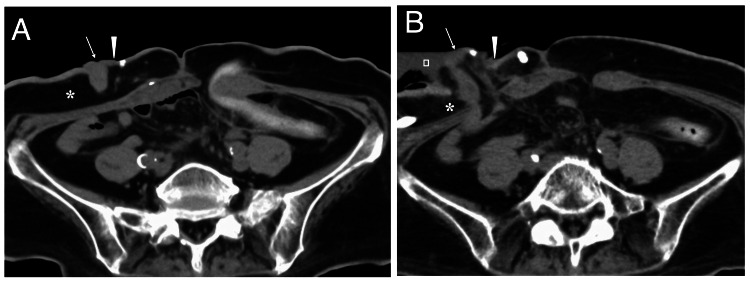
Computed tomography (CT) of and around the ileostoma A: CT showed that subcutaneous fat was thick as 3 cm (asterisk), and the top of the ileostoma (arrow) was located at the same level of the ileostoma base (arrowhead) and the surrounding skin before reoperation; B: although watery feces (open square) partially obscured the ileostoma contour, CT showed the ileostoma (arrow) was much higher than the ileostoma base (arrowhead). Subcutaneous fat around the ileostoma (asterisk) was thinner than that before reoperation.
